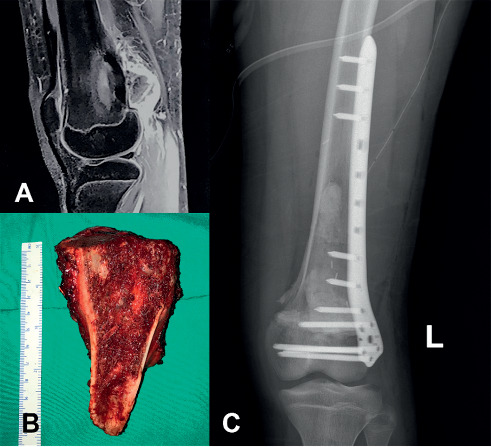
A sagittal T1-weighted fat-saturated MR image of a 14-year-old boy (case number 5) (a) demonstrating a broad-based tumour on the metaphyseal region of the distal femur. The patient was treated with hemicortical resection and prophylactic fixation using the plate and screw. Intraoperative gross pathology of the tumour (b) and postoperative radiograph (c) are presented.
